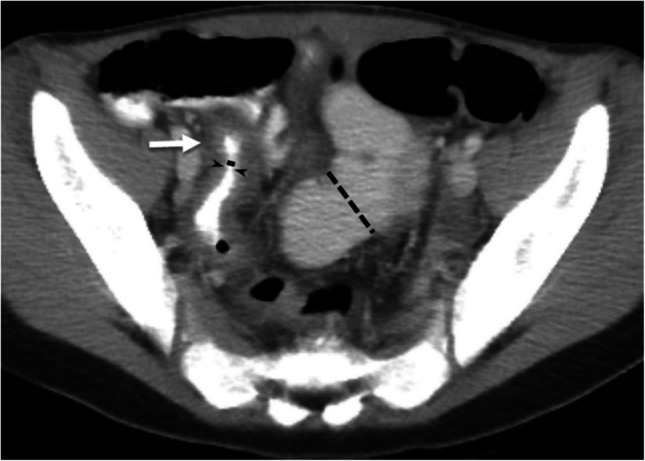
Example of terminal ileum measurements in a patient with luminal narrowing. A 13-year-old with Crohn’s disease. Axial CT image with IV and oral contrast material which demonstrates luminal narrowing and wall thickening of the terminal ileum. The minimum luminal diameter was approximately 1 mm (*solid black line*) while there was proximal terminal ileum maximal dilation of 28 mm (*black dash line*) which generated a luminal narrowing ratio of 28. The maximal wall thickness was 7 mm measured at the level of the *white arrow*
